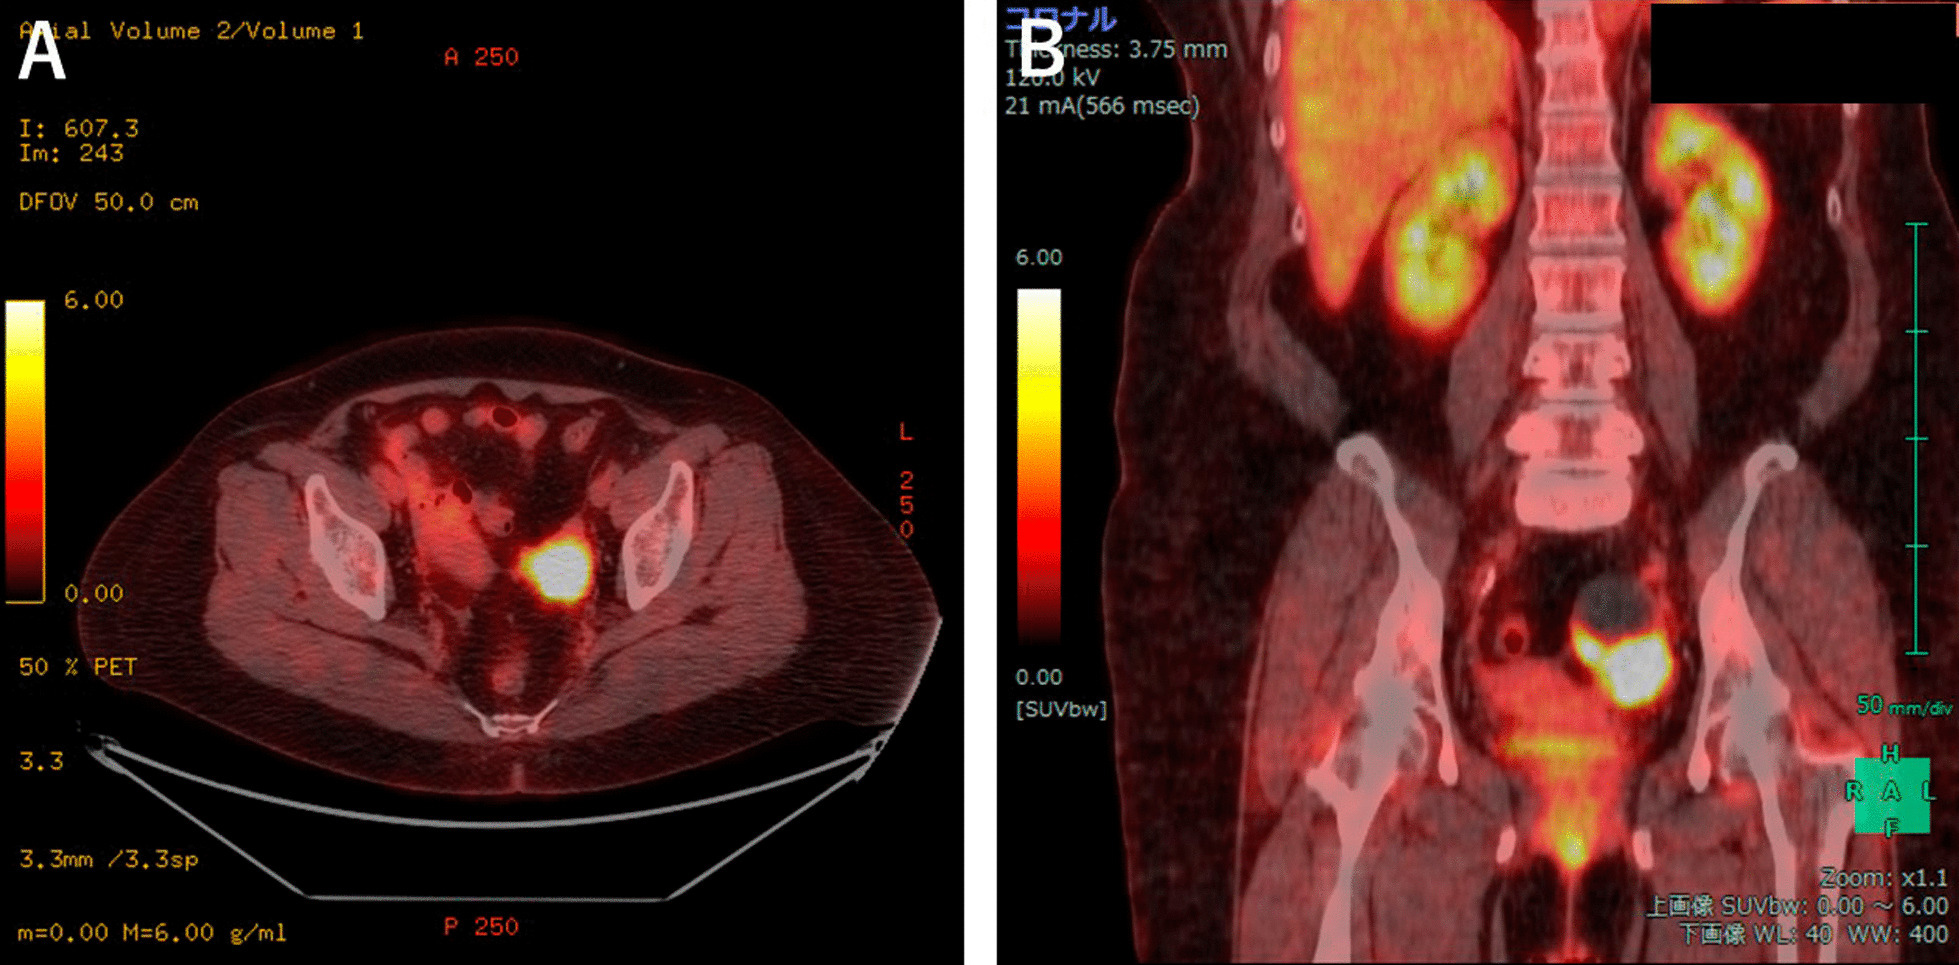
PET/CT scan of a mass in the pelvis. A PET/CT fusion image; and B coronal image. *PET/CT* positron emission tomography/computed tomography
